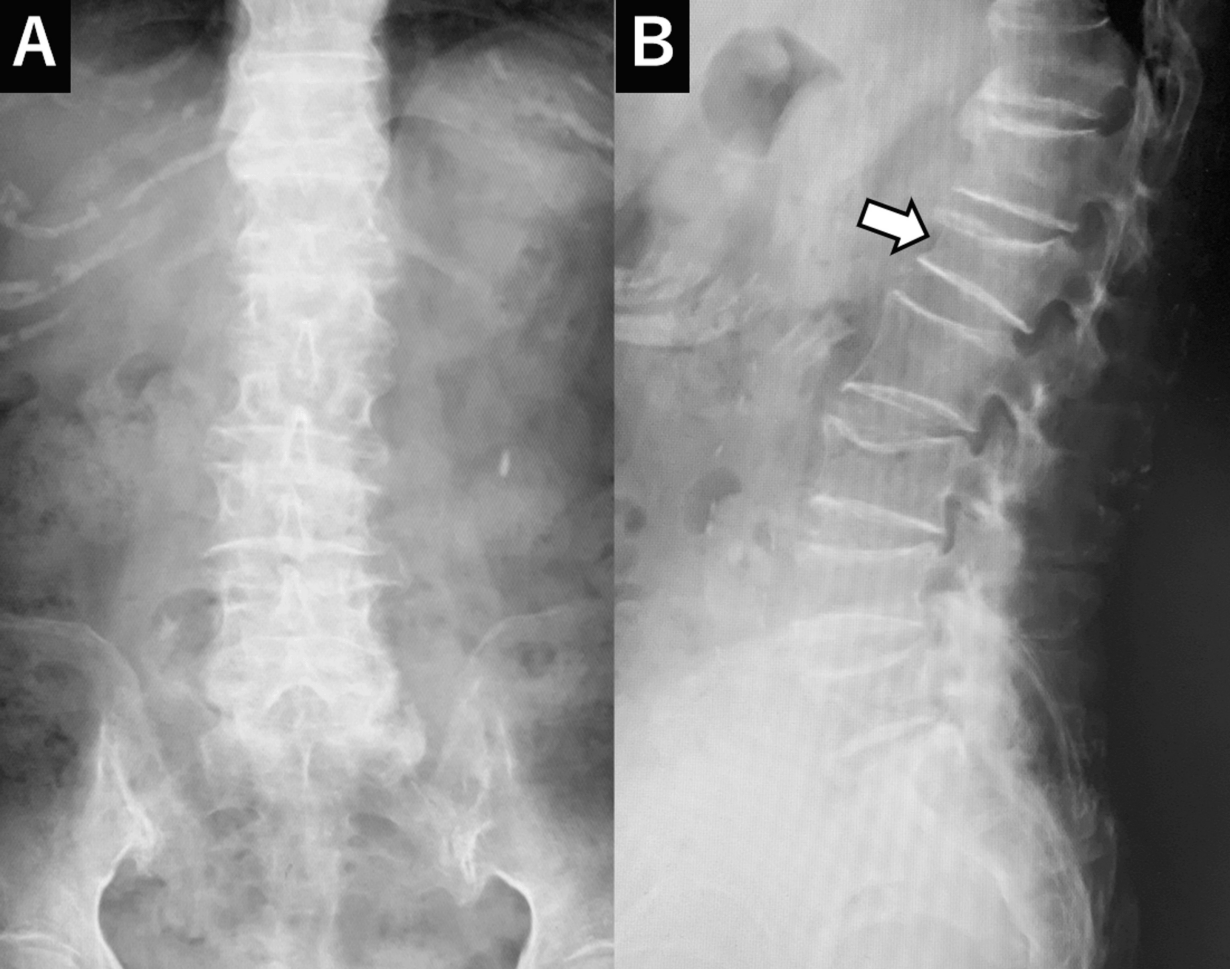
Initial X-ray An initial X-ray reveals a vertebral compression fracture at L1 (arrow).
